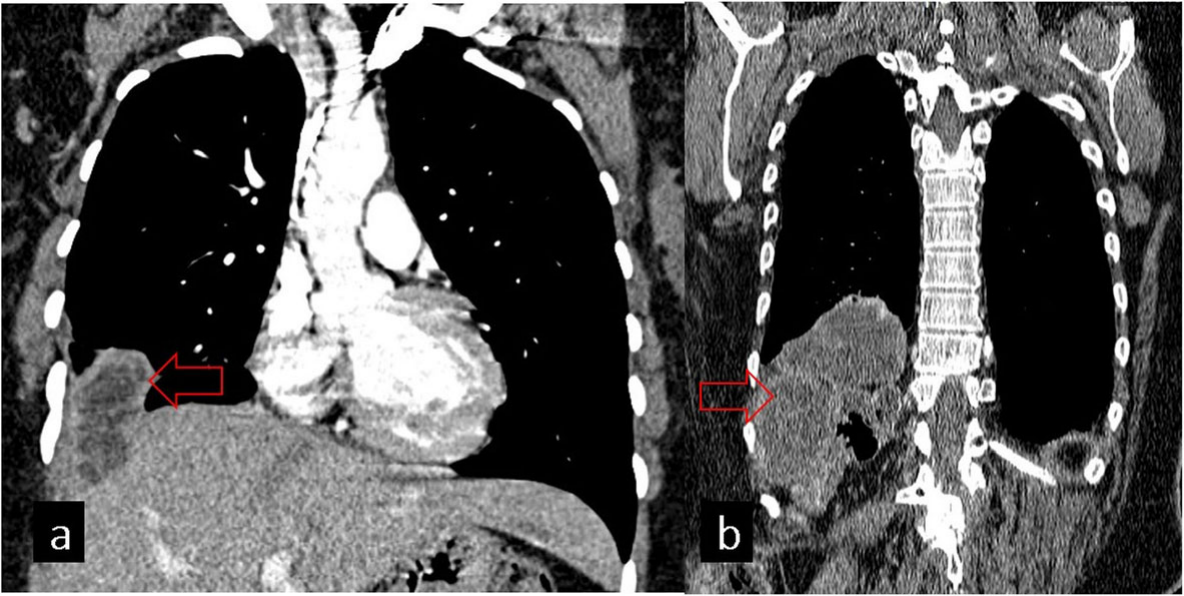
Chest CT images on the coronal plane show transdiaphragmatic migration of hydatid cysts in different patients (a, b, red arrows). Diaphragmatic discontinuation can be observed
